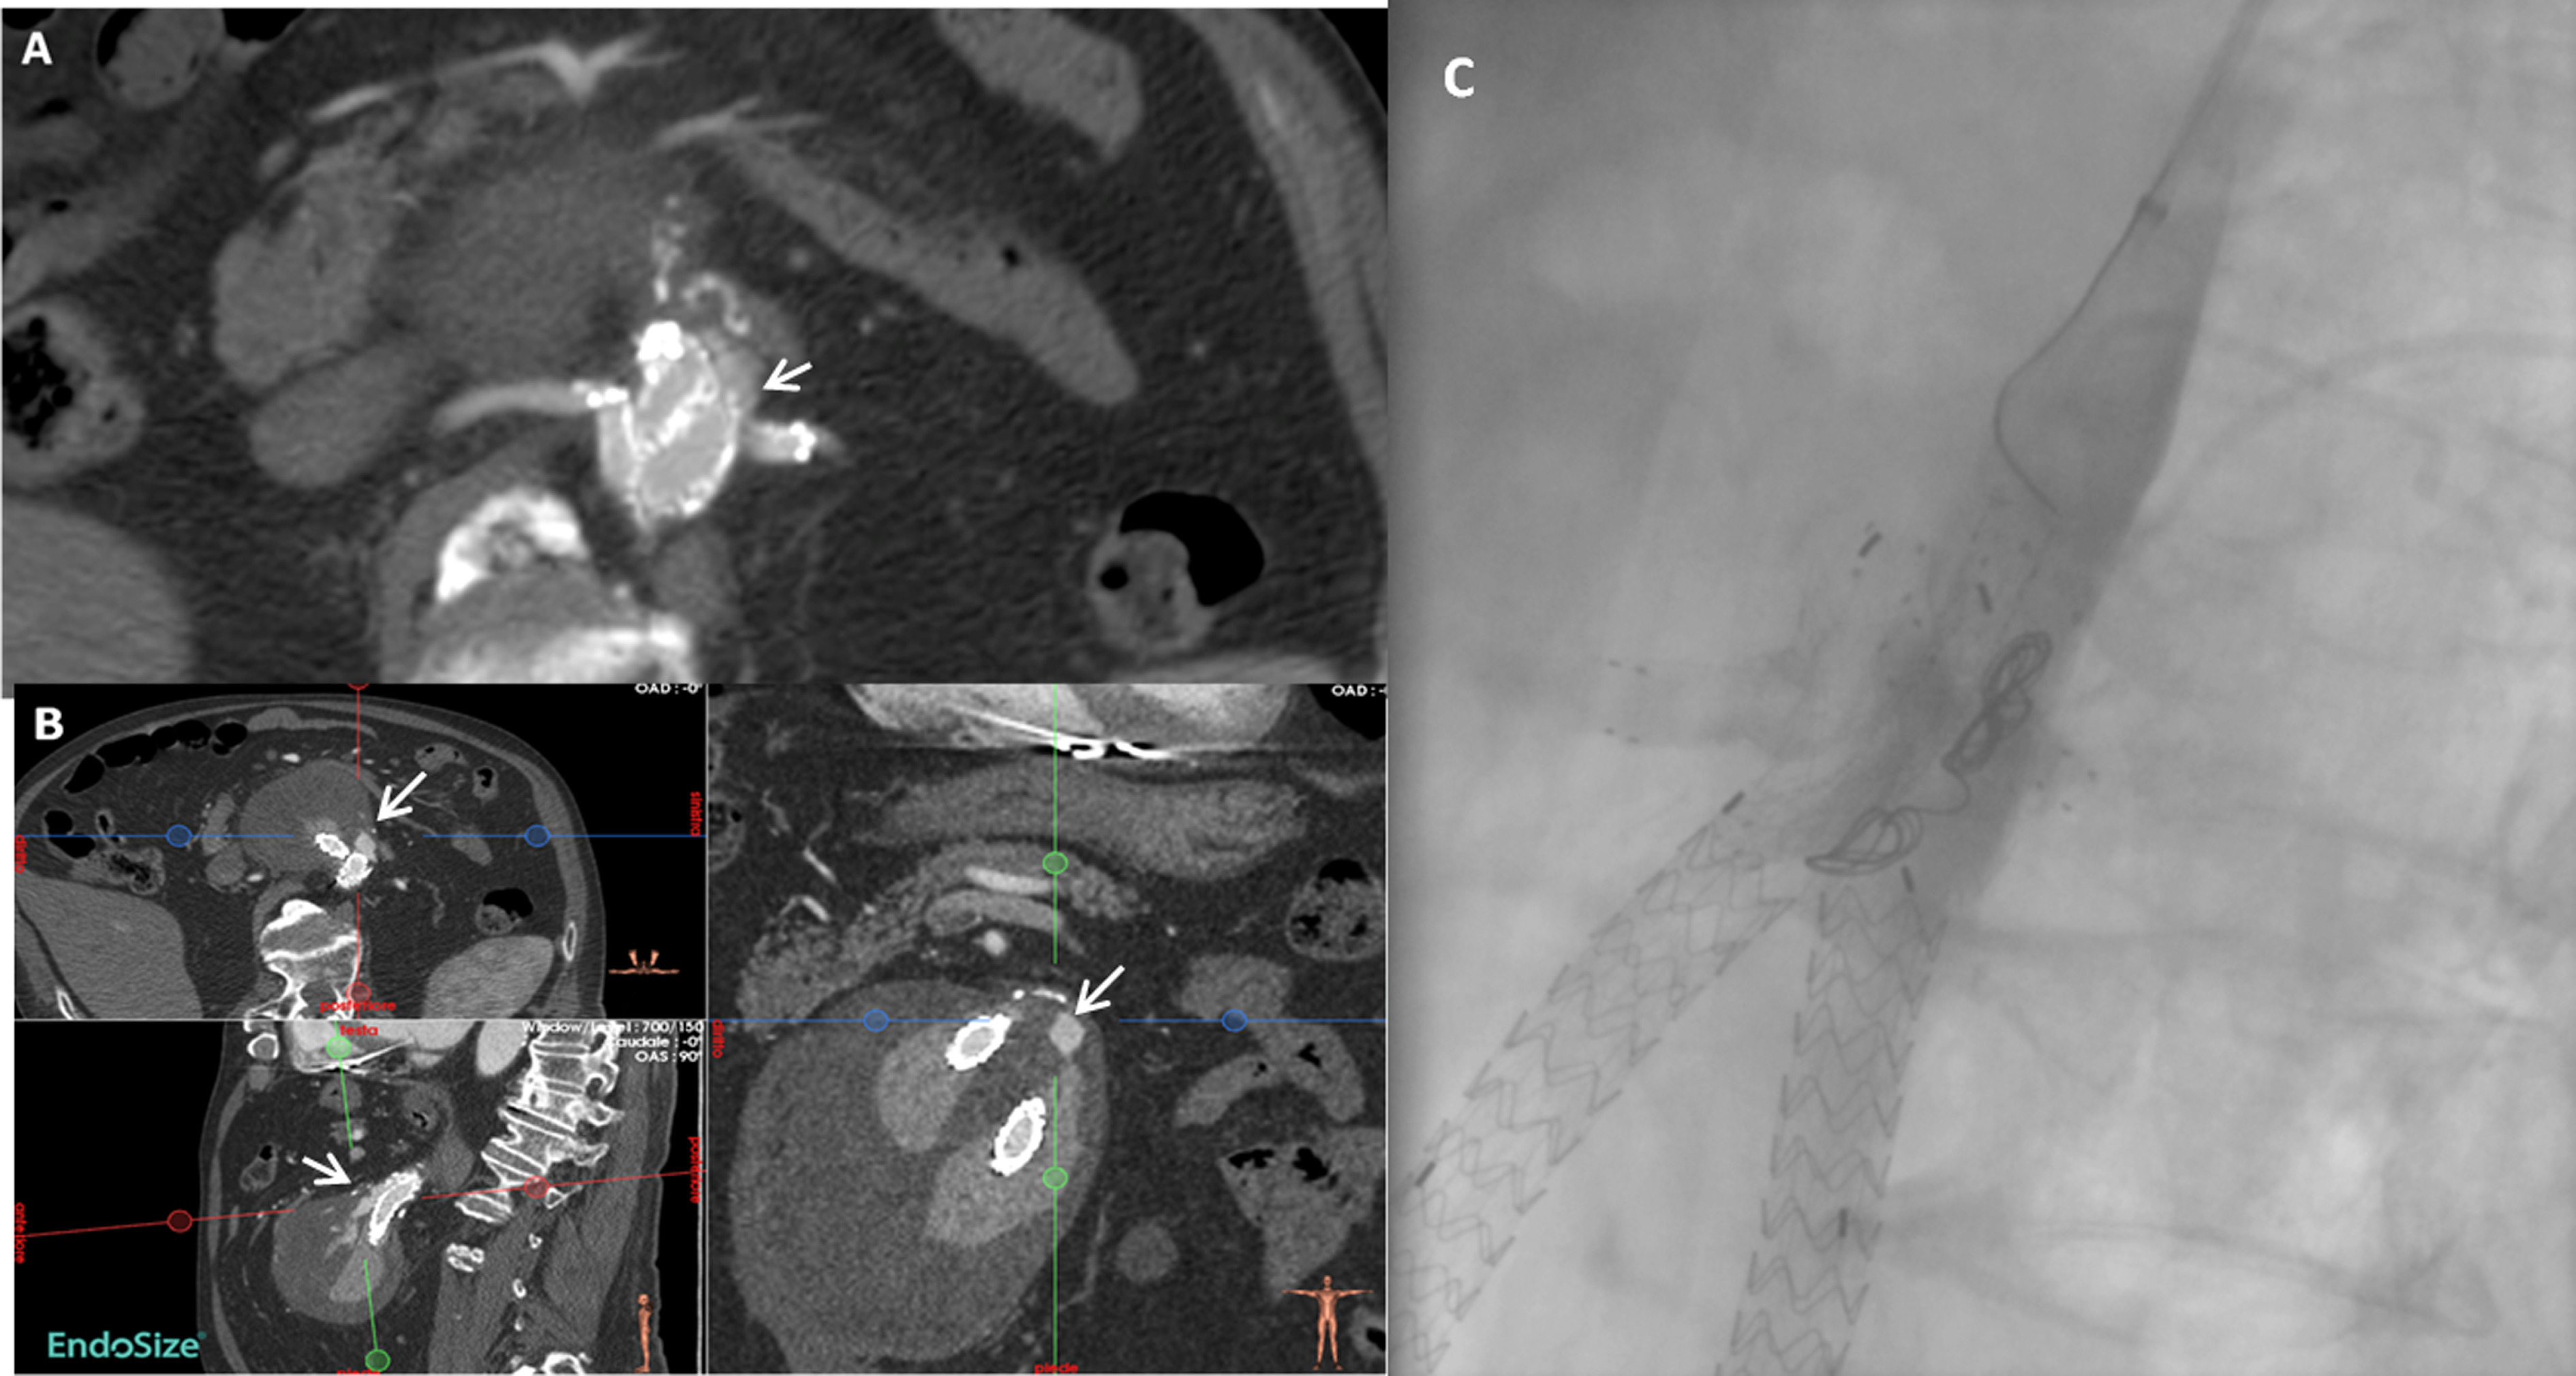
Fig. 3 Axial computed tomography (CT) image showing contrast medium between left renal artery stent and the two covered aortic stents after ChEVAS and proximal extension (A); persistent type Ia endoleak in different CT image views (B). Intraoperative angiographic image showing the final result after gutter embolization through coils (C).
